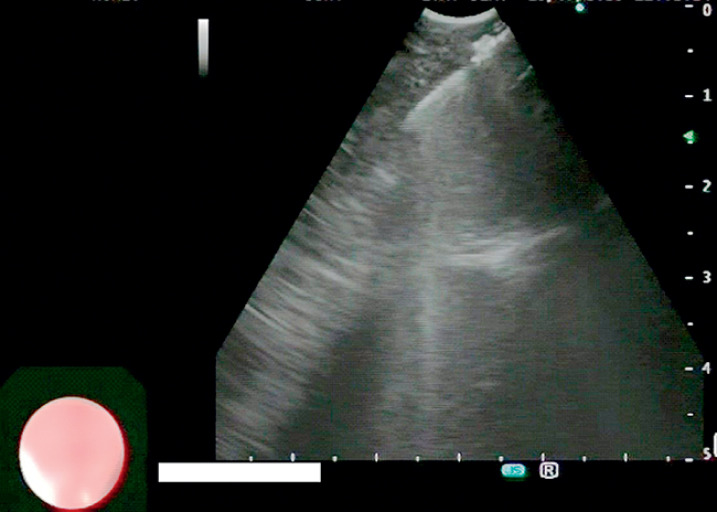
Transesophageal endoscopic ultrasound image with bronchoscope-guided fine-needle biopsy in a 6-year-old liver transplant recipient.
